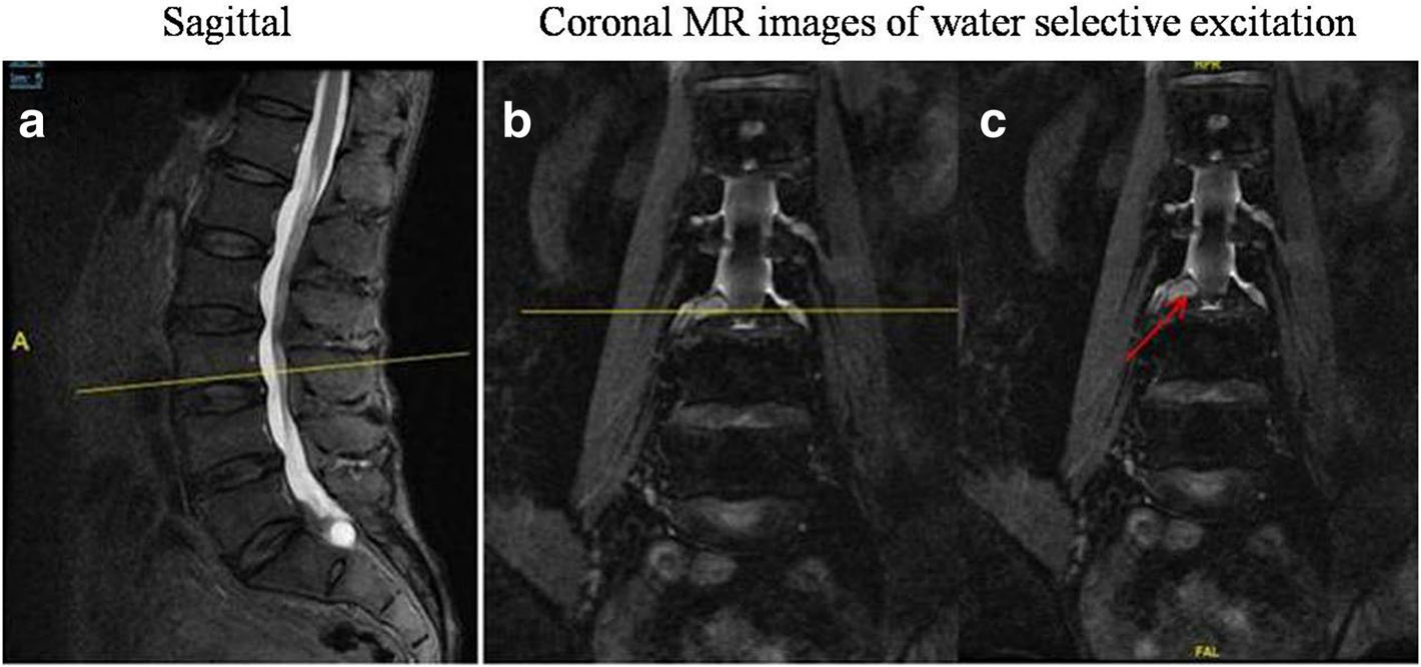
Coronal MR images of 3D fast-field echo with water selective excitation. T2-weighted MRI in the sagittal and axial plane was used to aid in localization (a and b). A clear boundary was observed between the mass and nerve root by coronal MRI (c)
